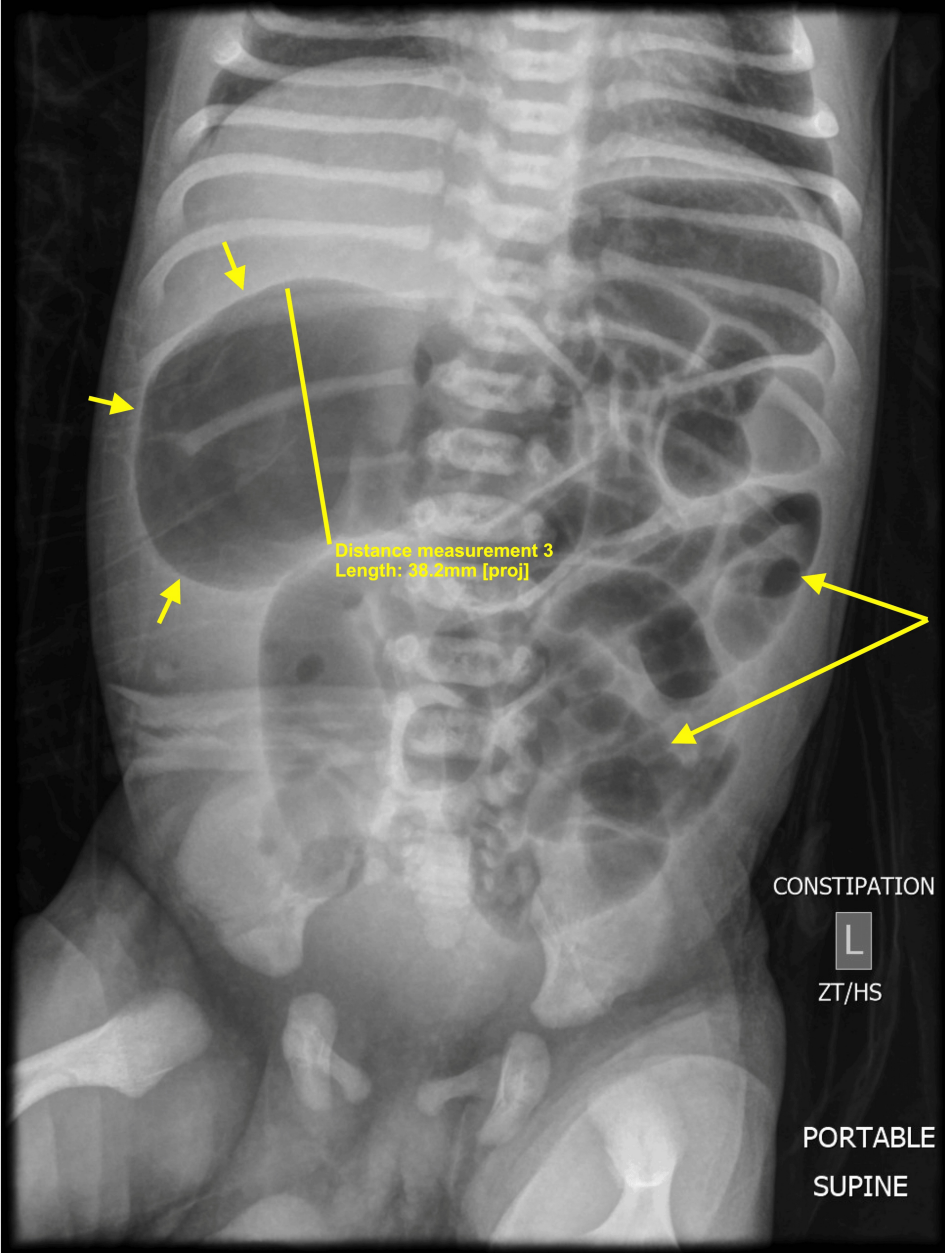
Abdominal radiograph shows significant gaseous distention (small arrows) and dilatation of colonic loops with prominence of small bowel gaseous distention (long arrows).
